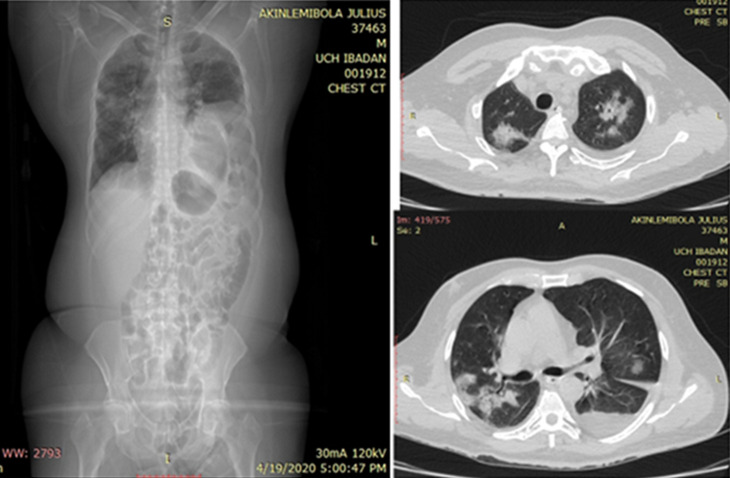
anteroposterior chest radiograph showing dextrocardia and features of bilateral pneumonic consolidations with peripheral disposition and an age-related aortic atherosclerotic calcification
